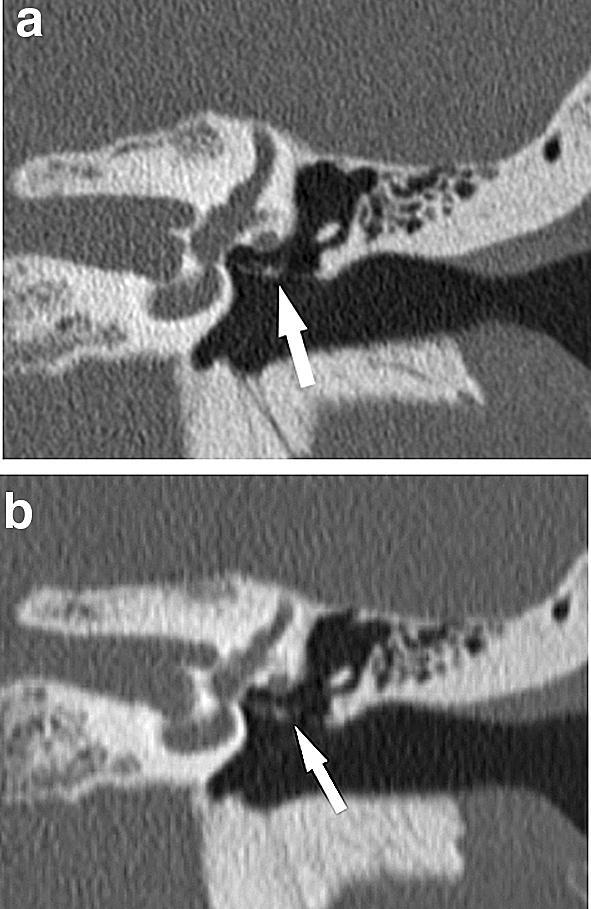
Coronal images of the left temporal bone CT from the same case. Image quality scores of the incudostapedial joint (arrow) are five on UHRCT (a), and three on MDCT (b) by both Reviewers
